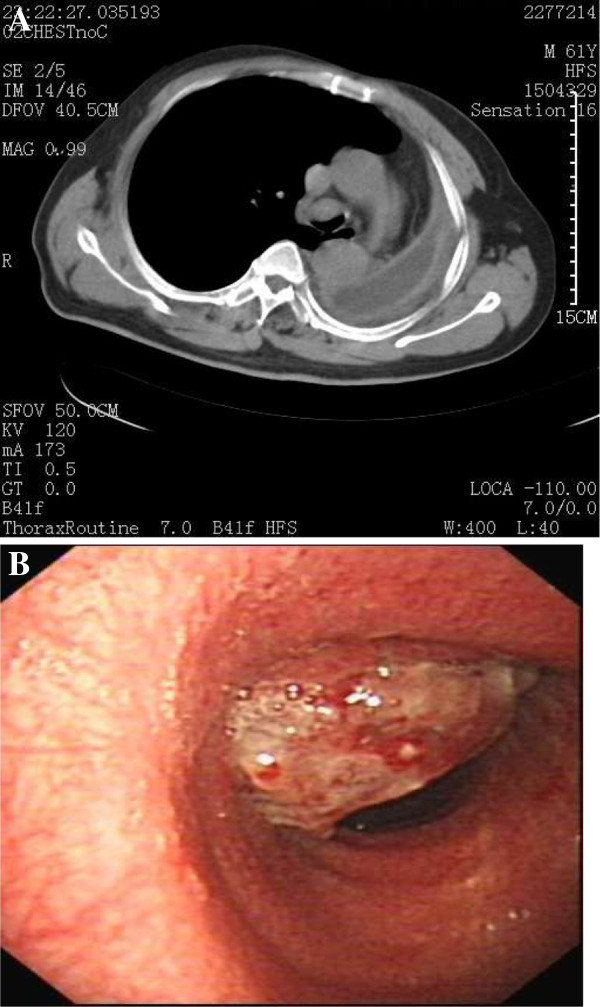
Scans of the patient’s chest show the mass in the end of the trachea. (A) Computed tomography scan. (B) Bronchoscopy.
